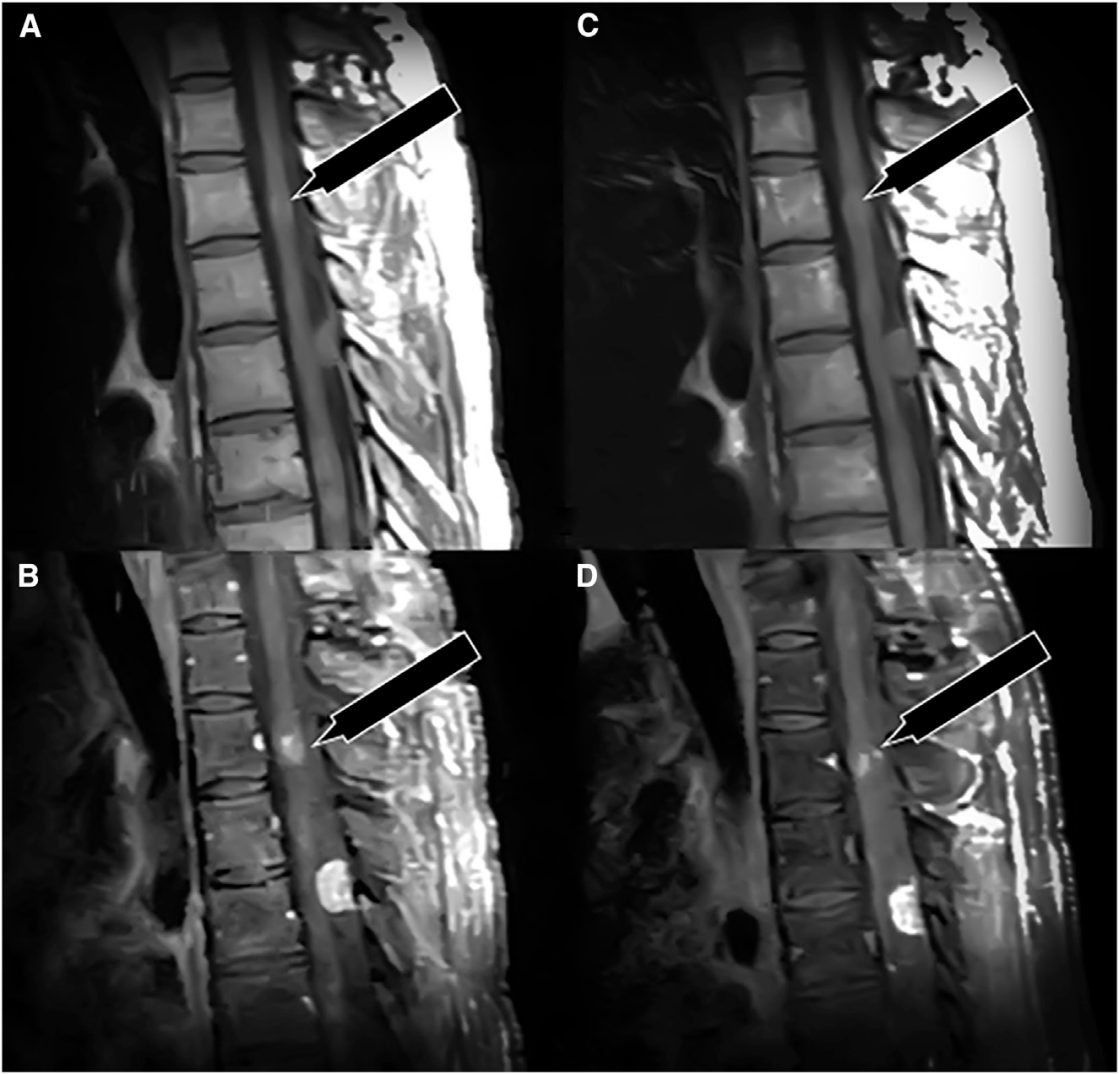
Response to 3-month selumetinib treatment Pre- (A, B) and post-treatment (C, D) thoracic spine MRIs at 3 months. (A) Pre-treatment non-contrast sagittal T1-weighted images of the thoracic spine demonstrate multifocal spinal cord signal alteration and subtle contour abnormality at the level of T3. An extramedullary posterior spinal canal lesion at the level of T5 is also seen. (B) Same-date sagittal T1 fat-saturation post-contrast images reveal avid enhancement in these tumors. The intramedullary tumors, including the T3 level lesion, are consistent with spinal ependymomas. The extramedullary T5 lesion with dural tail has imaging characteristics of meningioma. (C) Post-treatment non-contrast images taken at 3 months of treatment demonstrate similar distribution and characteristics of these same tumors. (D) Note the visible decrease in conspicuity of the T3 ependymoma, correlating with a measurable decrease in enhancement compared to pre-treatment images, compatible with a partial response. The T5 lesion persistently enhances. Special note: contrasted MRIs were taken after intravenous administration of gadolinium-based contrast material. Images were acquired at the same institution on the same scanner utilizing the same protocol. Regions of interest were drawn to include the tumors at the same level in the midline covering 50% of the total area. Standardized comparison of pre- and post-treatment images was performed on licensed post-processing software. “Enhancement” was standardized as a change in intensity after contrast administration of greater than or equal to Δ15. The T3 ependymoma lesion prior to treatment minimally enhanced (pre- and post-contrast intensity difference = Δ20). On 3-month post-treatment images, the lesion does not meet the threshold for enhancement (Δ3). For comparison and internal control, the T5 meningioma demonstrates significant pre and post-treatment post-contrast enhancement (Δ232 and Δ202, respectively). Thus, we cautiously support our claim of partial response with reproducible data.
